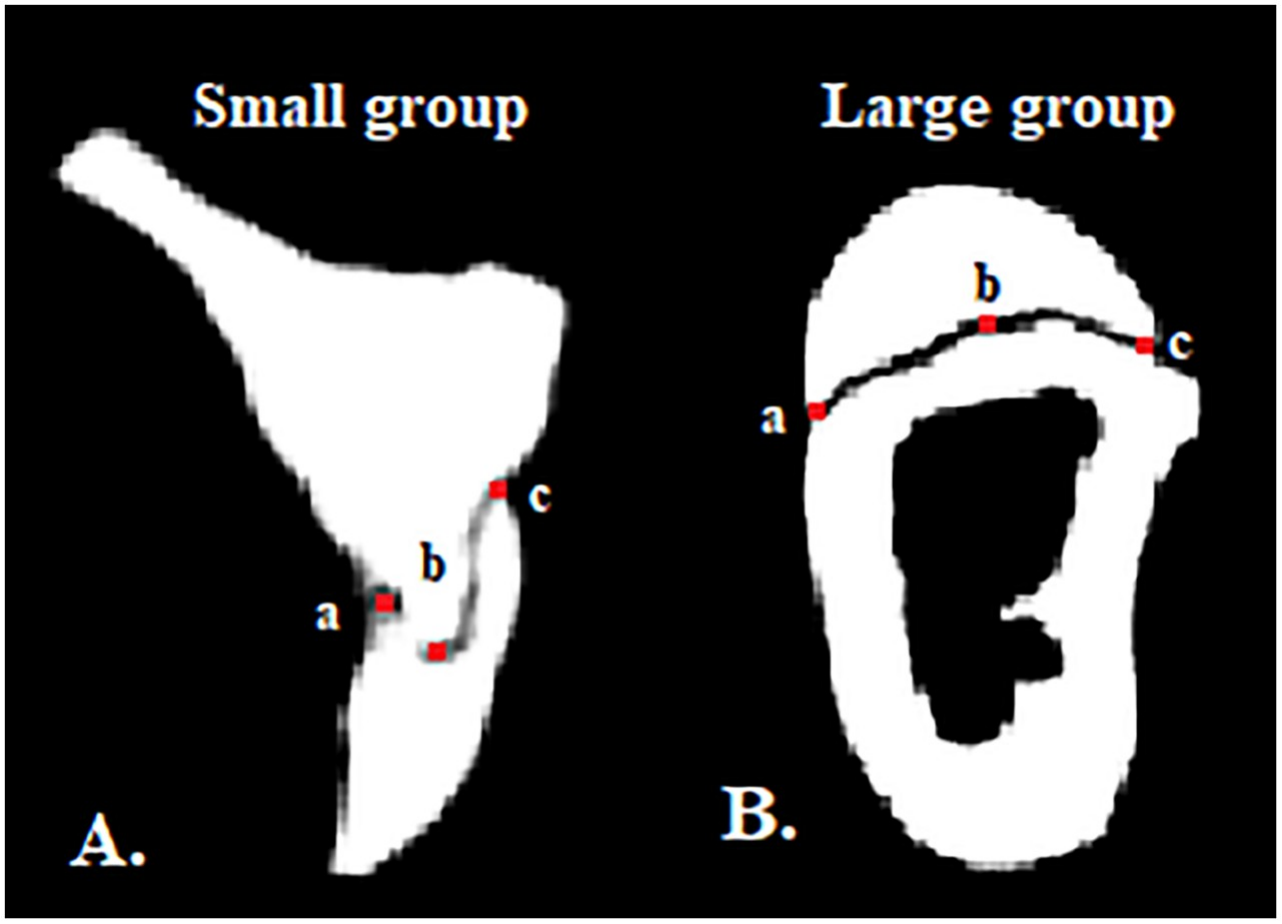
Cross-sectional slices of CBCT scans at the centre of the models with the fitted grafts. Representative slices depicting a small graft (A) and a large graft (B) fitted on the recipient defect sites of the alveolar ridge models; (a) lingual margin, (b) middle part and (c) buccal margin of the graft.
